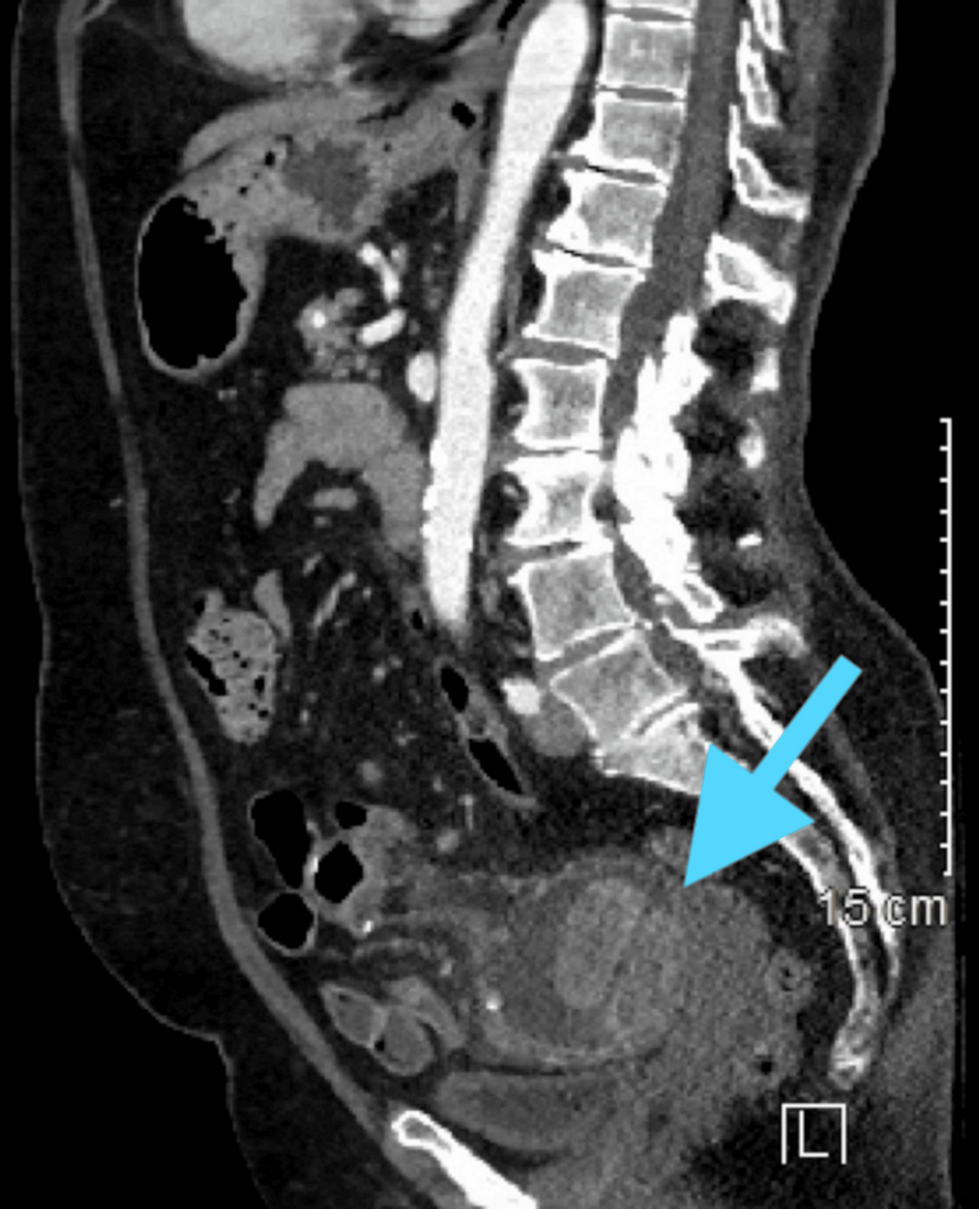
CT scan with IV contrast demonstrating retained material consistent with the enteroliths found intraoperatively.
